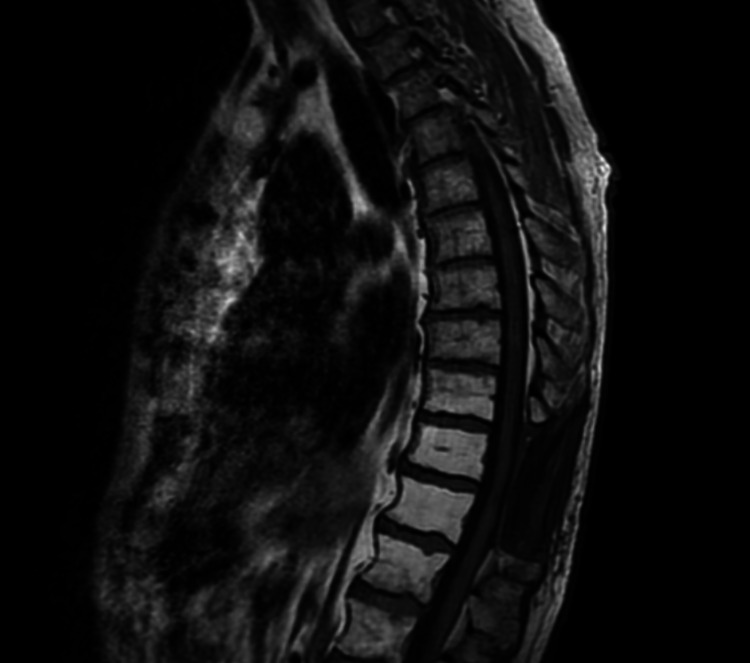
MRI of thoracic spine, T1 sagittal view. No evidence of disease recurrence.
